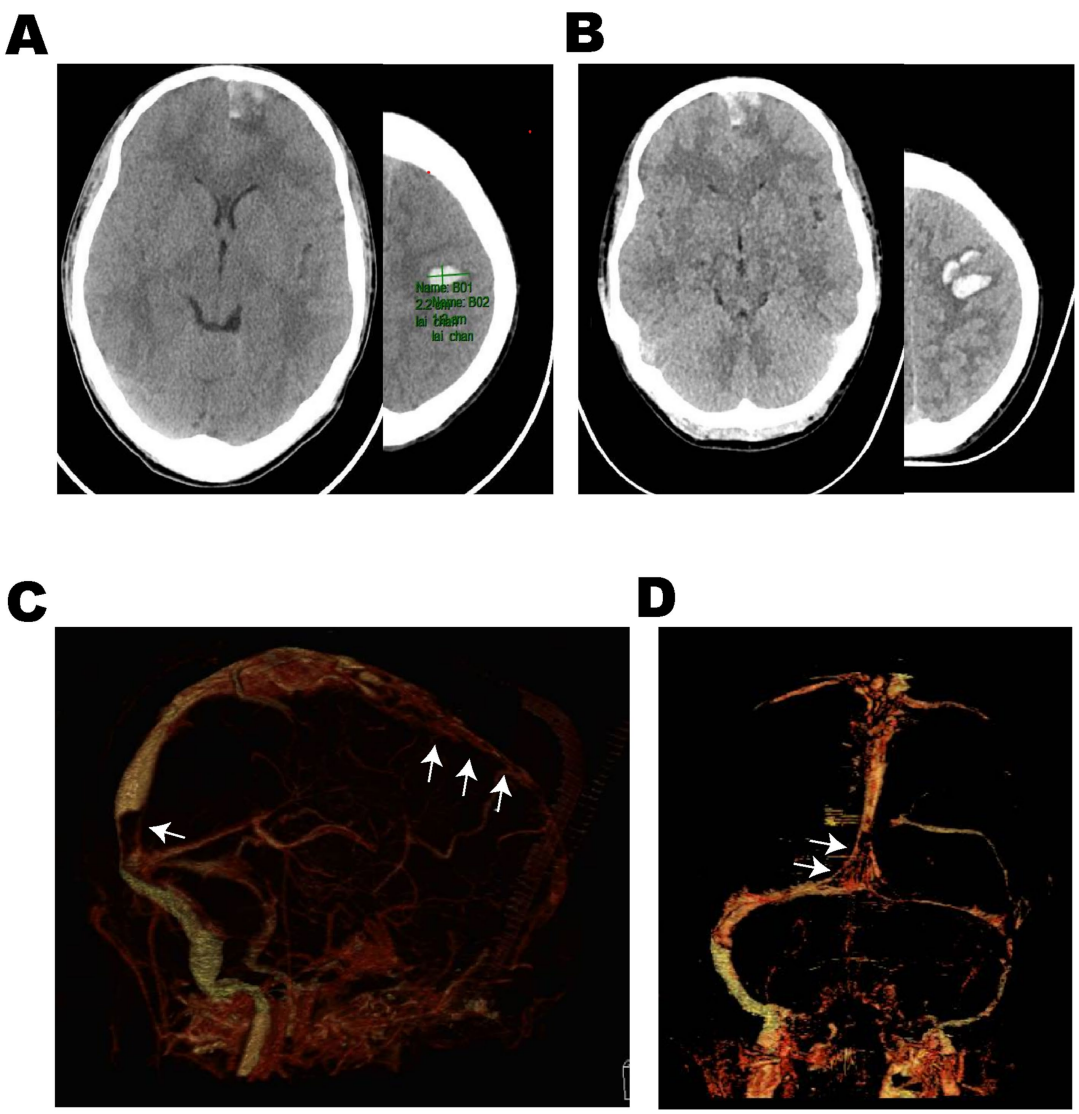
Case 8: An 18-year-old male adolescent was admitted with a 1-day history of sudden headache and limb convulsions. (A) Emergency head non-contrast CT scan (2023-May 28) showed multiple hematomas in the left frontal lobe. After low molecular weight anticoagulant therapy, dehydration and antiepileptic treatment, the mental state became worse, headache and limb convulsions could not be controlled. (B) The reexamination of head non-contrast CT (2023-May 30) showed the cerebral hemorrhage progressed. (C,D) Cranial CTV (2023-May 30) suggested multiple filling defects (White arrows) in the superior sagittal sinus and sinus confluence and the possibility of cerebral venous sinus thrombosis was considered. CTV computed tomography venography.
